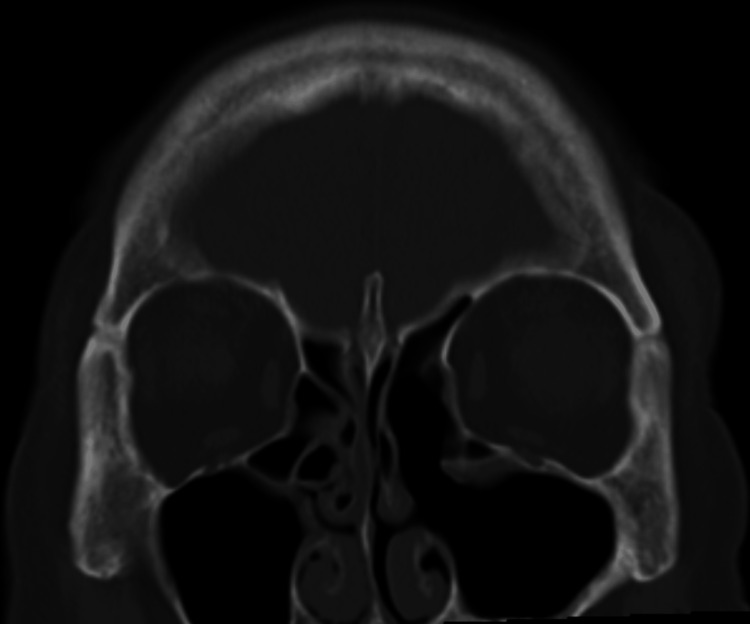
Coronal CT scan of the head performed eight months post-ESS for an unrelated issue showing that the left maxillary and ethmoid sinuses were clear, with no mucosal thickening or fluid retention, and good aeration. ESS: endoscopic sinus surgery
